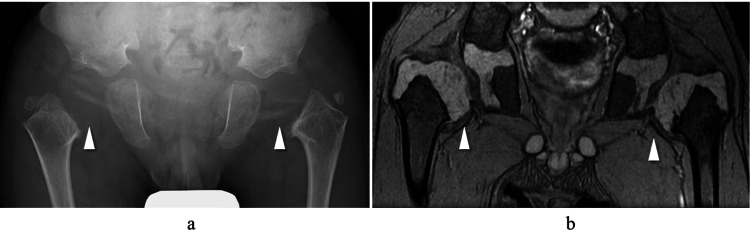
Plain radiograph and MRI image at age 4. (a) Plain radiograph: The ossification center is not visible (white arrowheads), and lateral displacement of the proximal femur is observed. (b) MRI T2*-weighted image: The femoral neck is shortened, and the femoral head is subluxated (white arrowheads).
